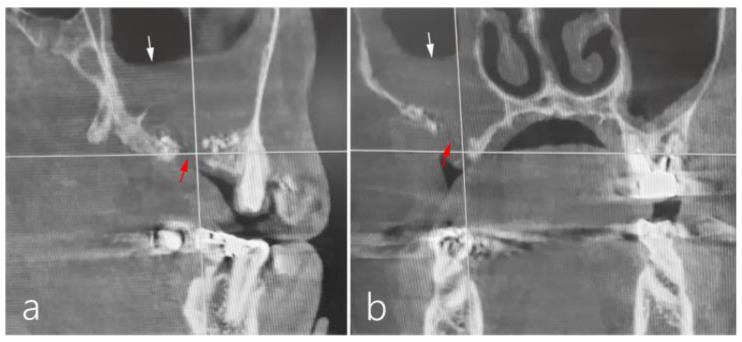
Case 3: (a) In the preoperative sagittal image of CBCT, the previous augmented bone graft had mostly disappeared, and there was severe sinus mucosal thickening (white arrow). (b) A bone defect extending from the ridge crest to the buccal side (red arrow) was observed on the preoperative coronal CBCT.
